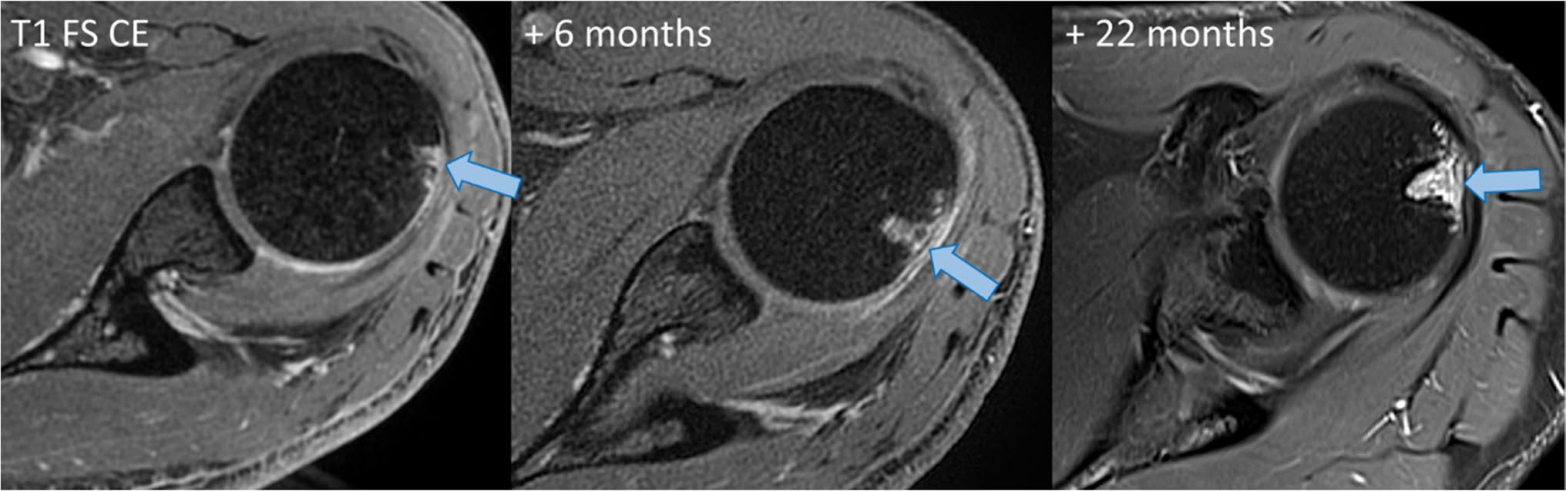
Evolution of greater tuberosity erosion on T1-weighted contrast-enhanced (CE) MRI from initial imaging to 6 months and an additional 22 months following initial MRI in a 25-year-old male, increase in the size of the greater tuberosity erosion (blue arrow) is shown
